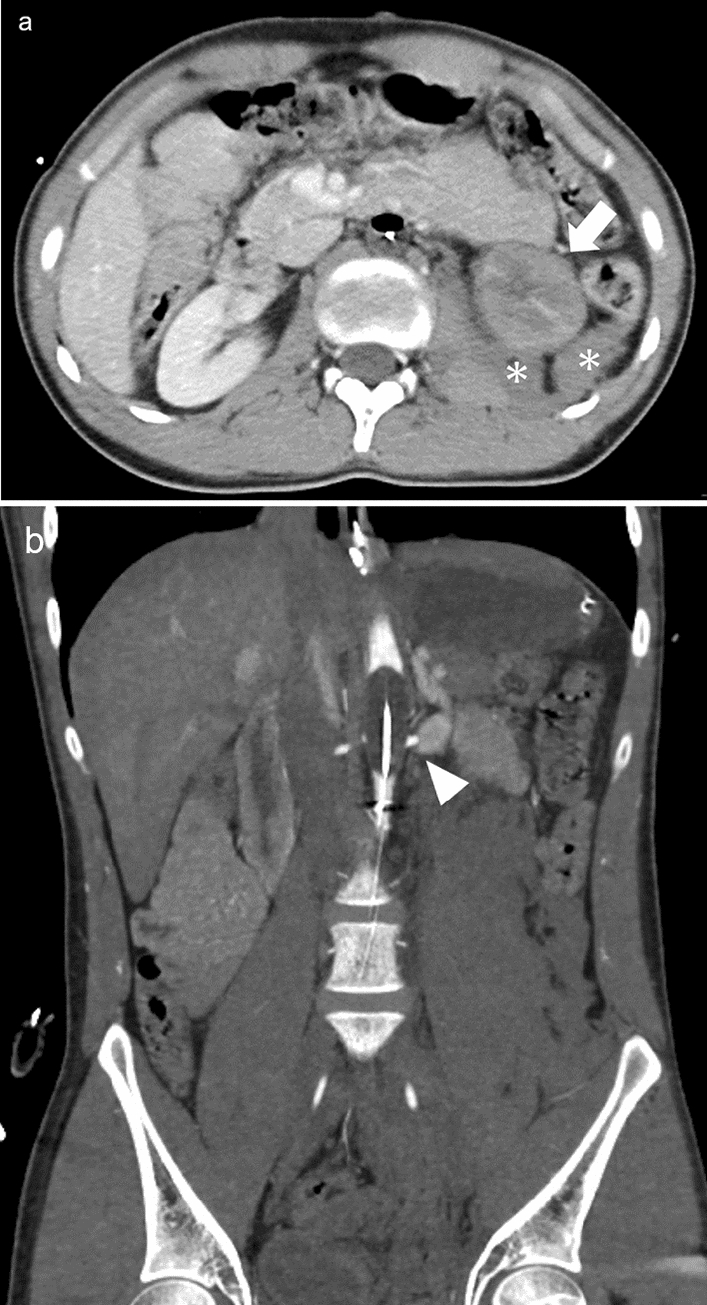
Abdominal contrast-enhancement computed tomography (a: axial image, b: coronal view) with complete resuscitative endovascular balloon occlusion of the aorta (REBOA) in a patient with multiple traumas showing decreased enhancement on the left kidney (a, arrow) and hematoma around it (a, asterisks), which is suggestive of renal contusion. However, in this case, REBOA was misplaced in Zone II and the orifice of the left renal artery was occluded by the balloon (b, arrowhead). Therefore, it is difficult to conclude that this case was REBOA-related hypoperfusion or renal injuries
